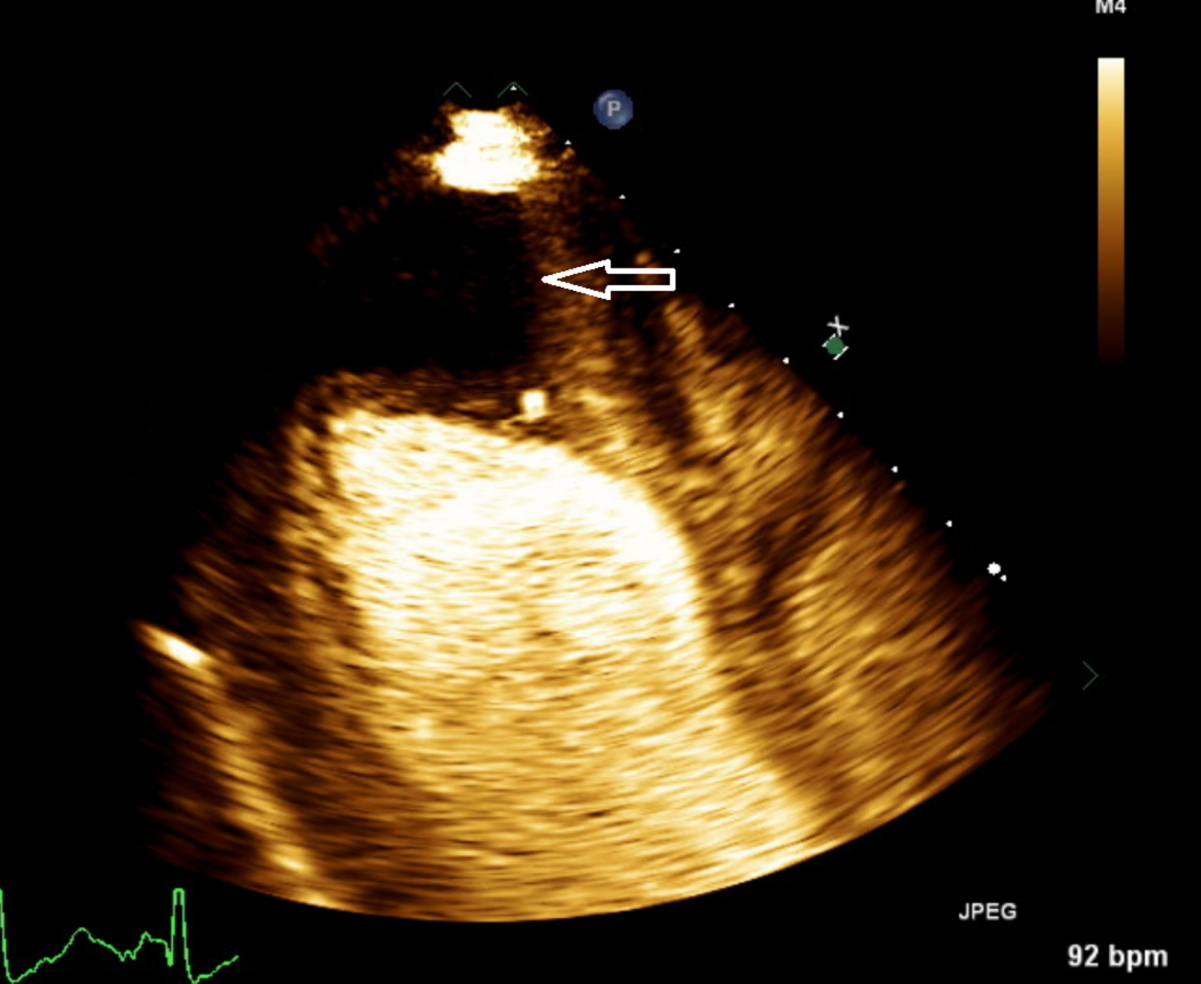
Patient 1 Echocardiogram
